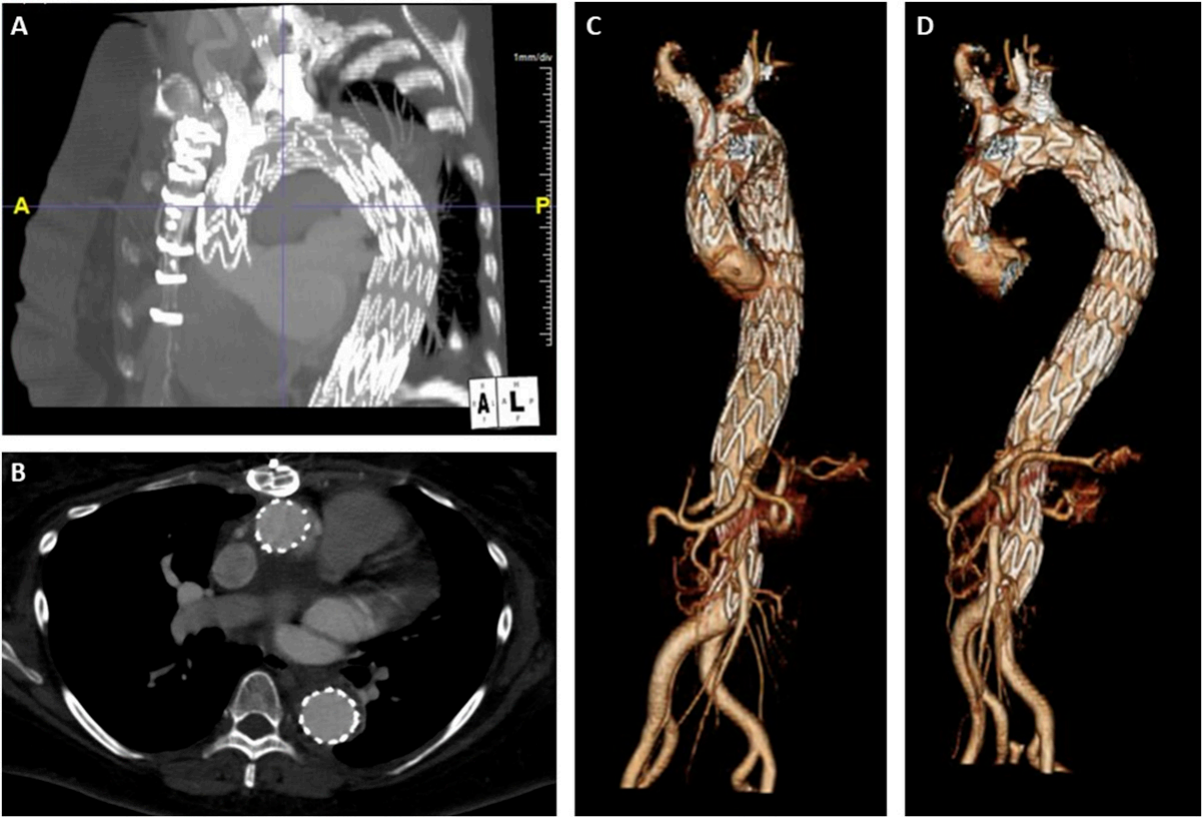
Sagittal (A) and axial (B) view of follow-up abdominal CTA with aneurysm sac shrinkage, PMEG vessel stent patency, and appropriate LSCA occlusion. Anterior (C) and anterolateral (D) 3D postoperative reconstruction of physician-modified endograft (PMEG) thoracic endovascular aortic repair (TEVAR).
